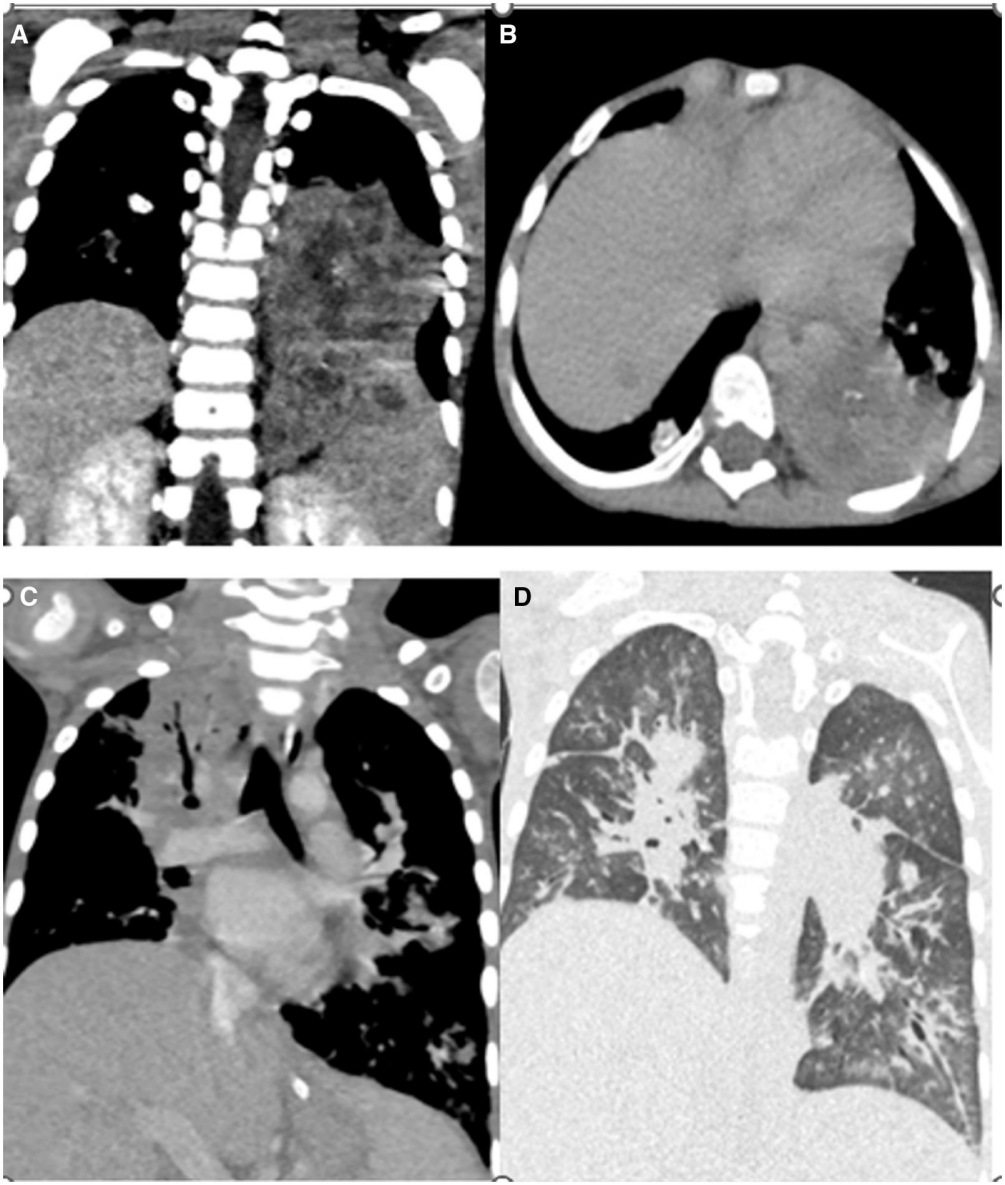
(A) Coronal and (B) axial post-contrast CT chest images showing a large heterogeneously enhancing parenchymal lesion in left lower lobe with areas of necrosis within causing complete occlusion of left lower lobe bronchus. (C) Coronal post contrast and (D) coronal CT chest images post corticosteroid therapy after 1 month showed complete resolution of left lower lobe lesion but showed increase in right perihilar infiltrates.
